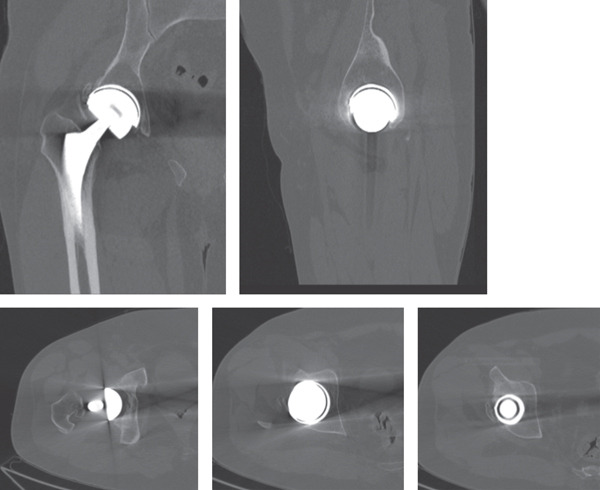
CT scan showing no signs of osteolysis and proper acetabular cup positioning.
